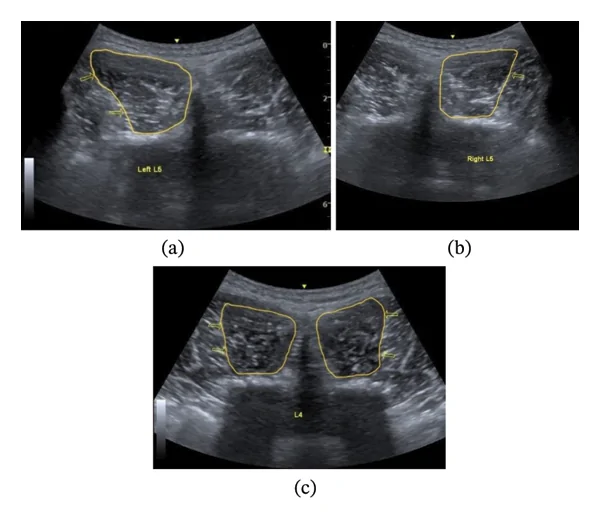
Ultrasound images of (a) left L_5_ multifidus cross‐sectional area, (b) right L_5_ multifidus cross‐sectional area and (c) bilateral L_4_ multifidus cross‐sectional area.
